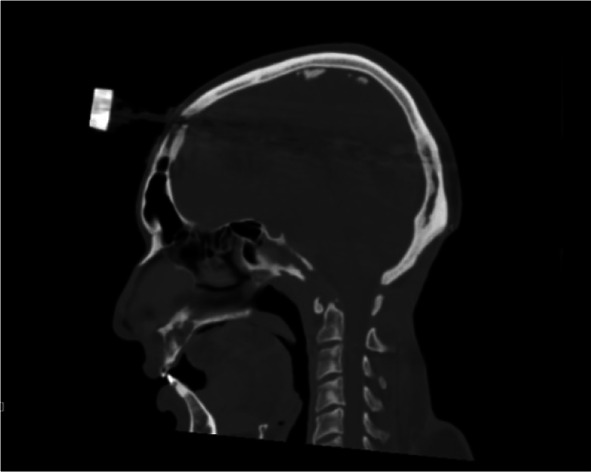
Post debridement CT showing eroded skullbase and atlas
